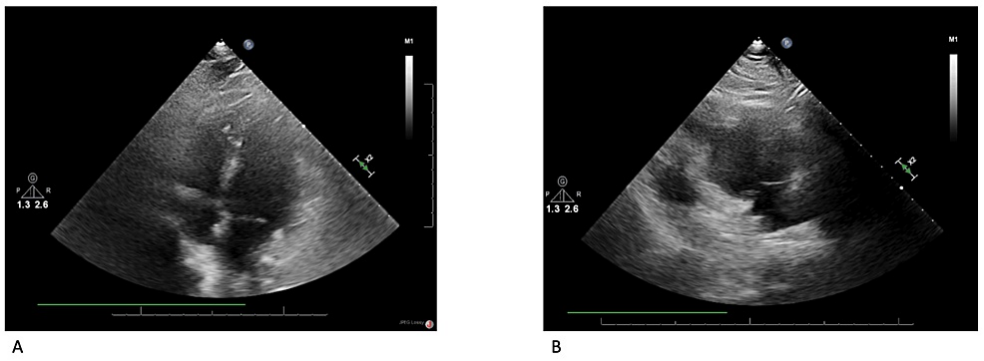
TTE Figure 1A depicts the TTE Apical 4-Chamber view. Figure 1B depicts the RV inflow view. The TV septal leaflet and the RV septum can be visualized in each view without any clear evidence of vegetation on the TV or in the RV. TTE: Transthoracic echocardiogram; RV: Right ventricle; TV: Tricuspid valve
